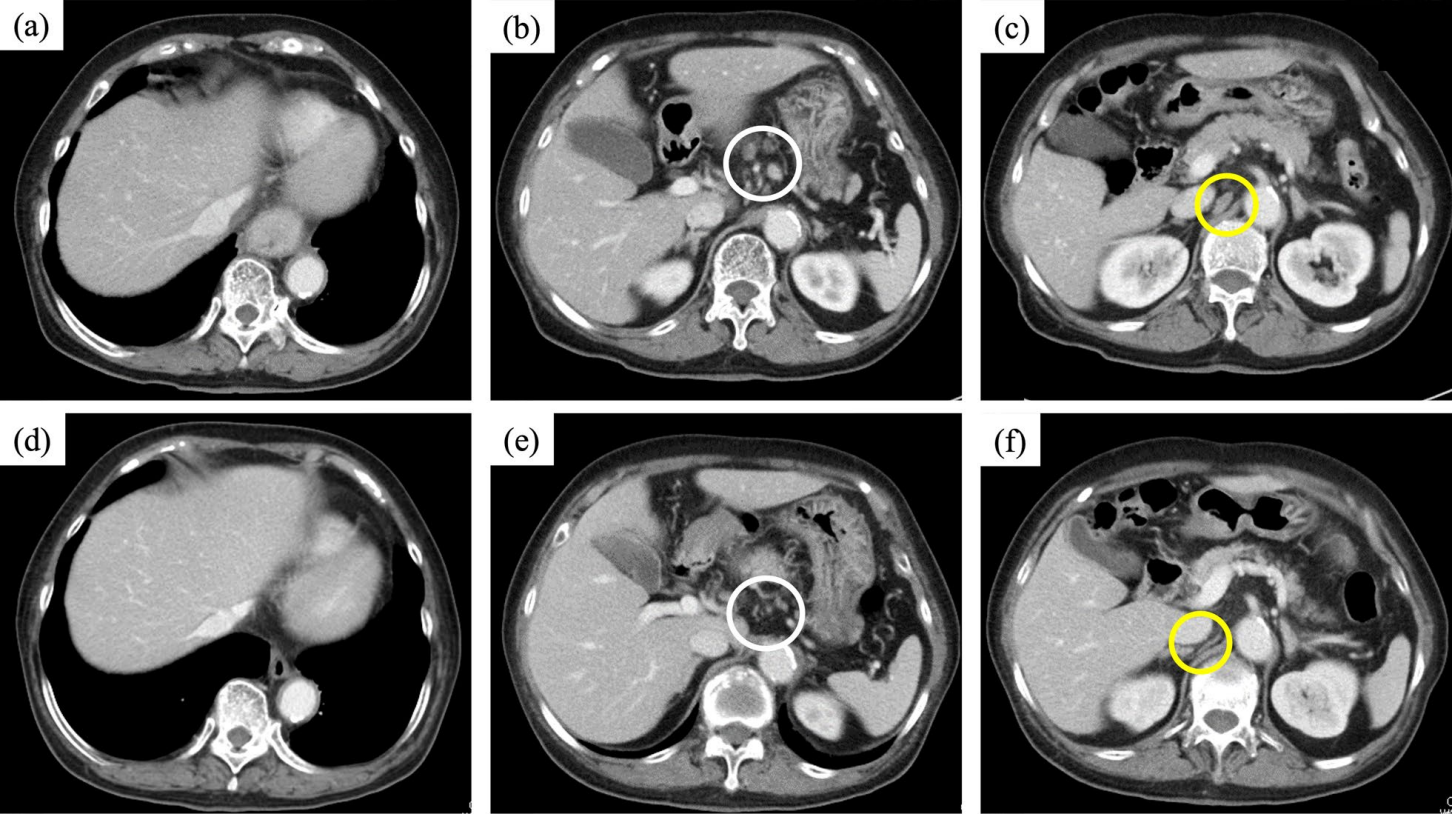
Enhanced computed tomography. Abdominal computed tomography reveals the thickening of the esophageal wall in the thoracic lower esophagus (a). The swelling of regional lymph nodes (b, white circle) and the lymph node on the dorsal surface of the inferior vena cava (c, yellow circle) is observed. Following preoperative treatment, the primary tumor (d), its regional lymph nodes (e, white circle), and the lymph node on the dorsal surface of the inferior vena cava (f, yellow circle) appear to shrink
